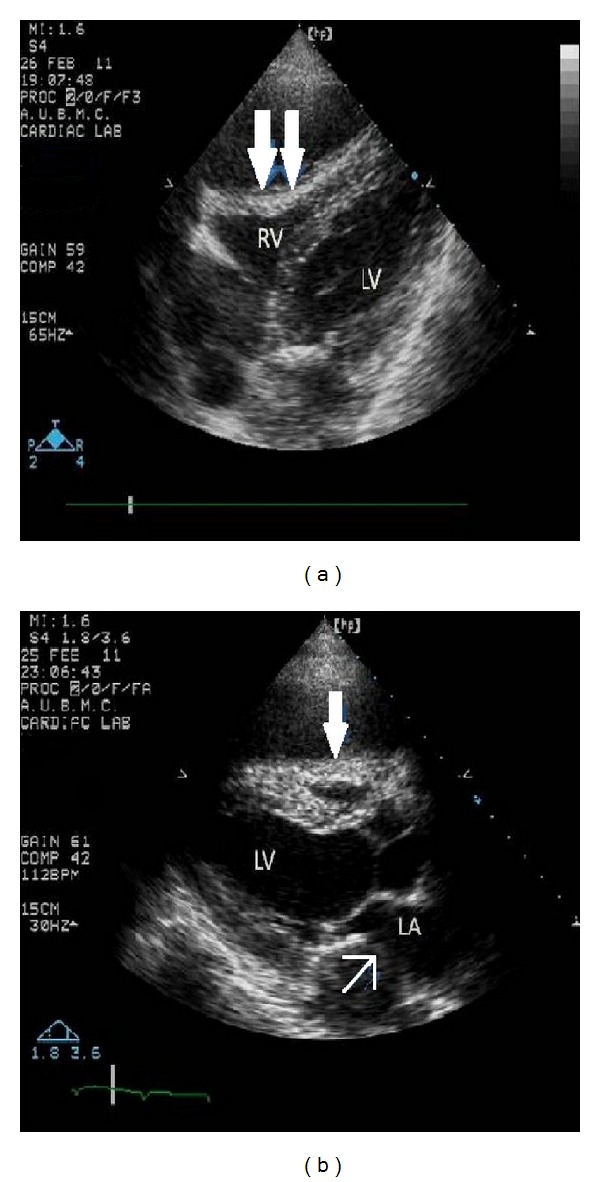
Echocardiography shows the cystic mass surrounding the heart (full arrows) with impingement on the right ventricle and left atrium.
